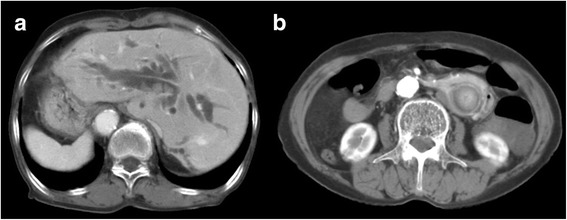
a Diffuse dilatation of biliary tree with multiple intrahepatic duct stones and CBD stones. b CT revealed situs inversus totalis
